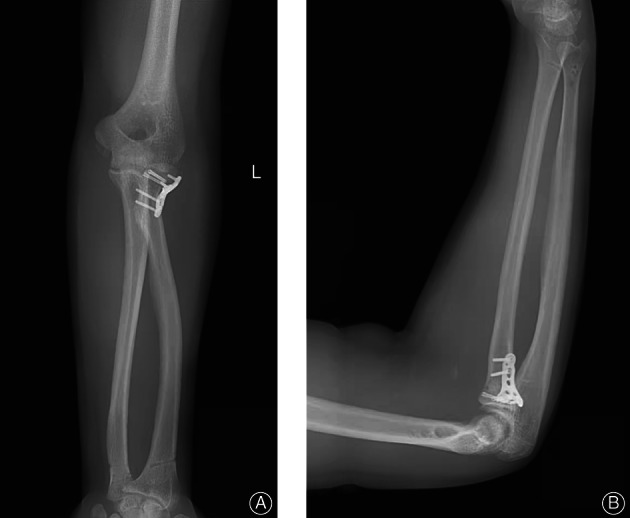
An ultra‐elbow and wrist plaster was used to flex the elbow by 90°, and the wrist was fixed in the supine position for 6 weeks. X‐rays were reexamined every week to monitor the fracture position. Orthopedic rehabilitation exercises were performed twice a week from 4 weeks to 6 months after the operation.(A, B). After 12 months of postoperative follow‐up, the patient was symptom‐free, and radiographs showed fracture healing
